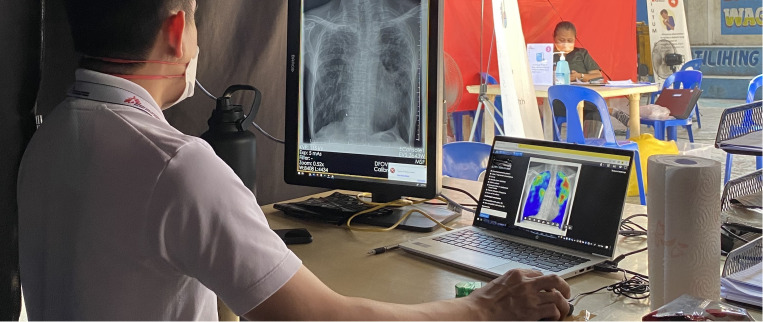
MSF physician using computer-aided detection (CAD) software to support chest X-ray interpretation during TB active case finding in Manila, Philippines.
